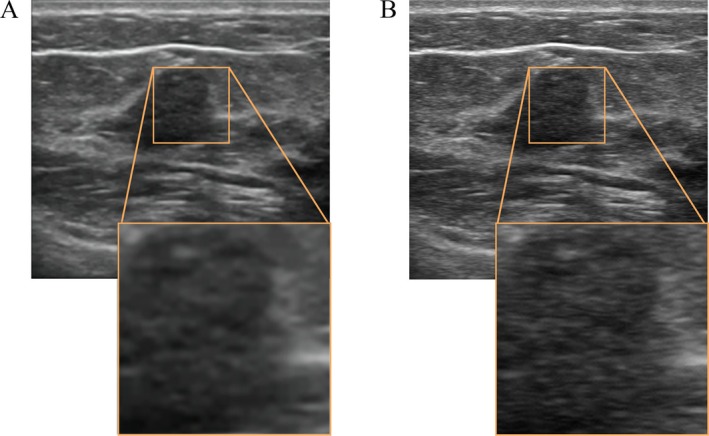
The representative original ultrasound images and their corresponding super‐resolution images from a 43‐year‐old female with pure ductal carcinoma in situ. Original high‐resolution ultrasound images (A) is less blurry compared to super‐resolution images (B) as shown in the orange square box.
